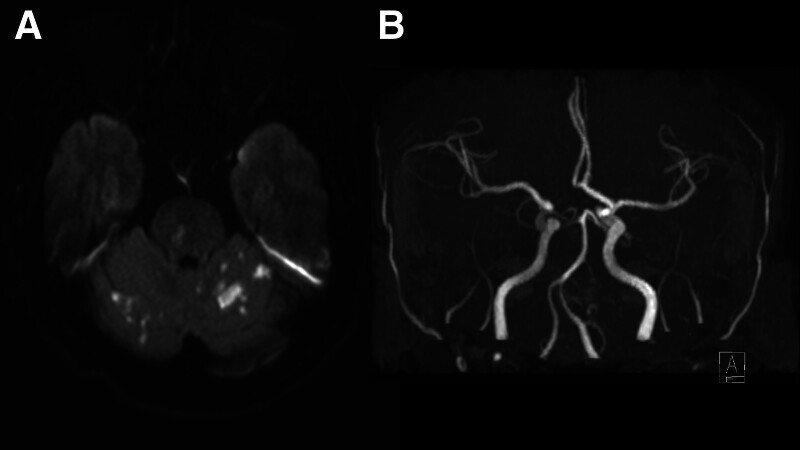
Diffusion-weighted MR imaging revealing multiple recent infarctions in the posterior circulation involving bilateral cerebellum and pons (A); MR angiography showing no evidence of stenosis of the major intracranial arteries (B). MR = magnetic resonance.
